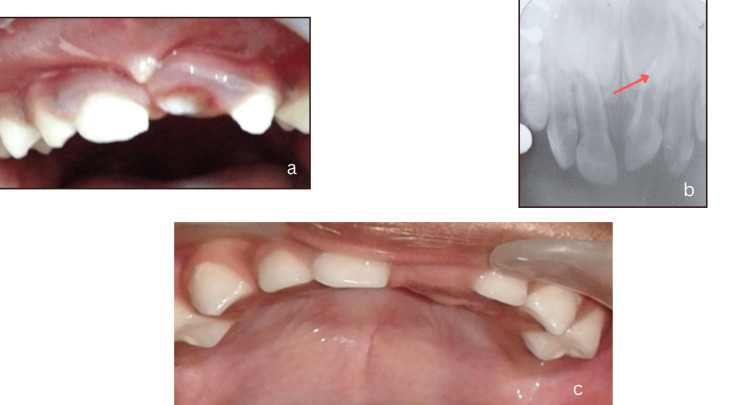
(a) Intra-oral examination during the first visit of the child patient at two years of age revealing the displaced 61 with only incisal edge clinically visible. (b) Intra-oral periapical radiograph revealing severe intrusive luxation injury in 61 as indicated by a red arrow line. (c) Post-operative image after six months following extraction of 61.
